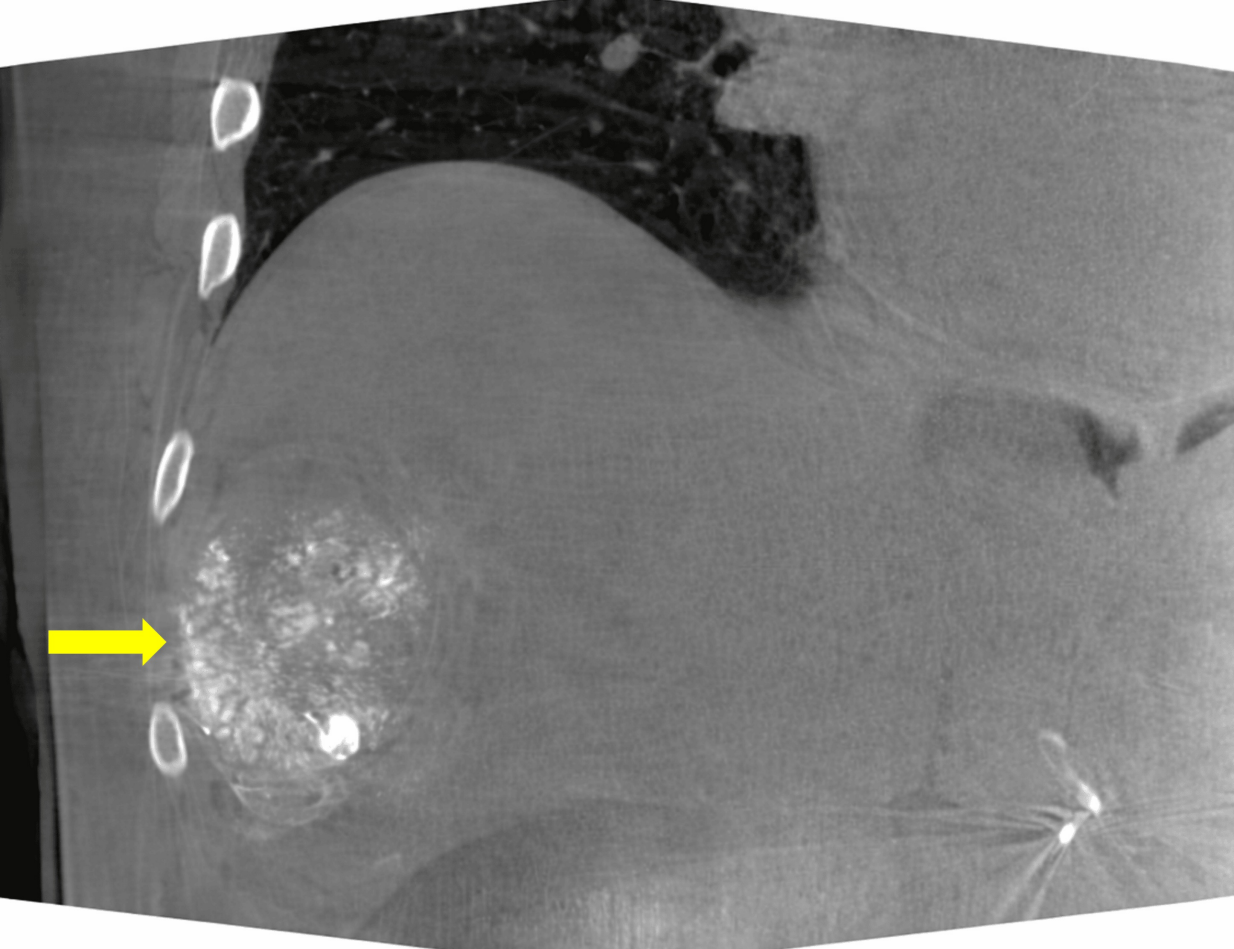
Coronal contrast-enhanced computed tomography (CT) image demonstrating a residual lesion (yellow arrow) in hepatic segment V containing radiopaque material (lipiodol) after transarterial chemoembolization
